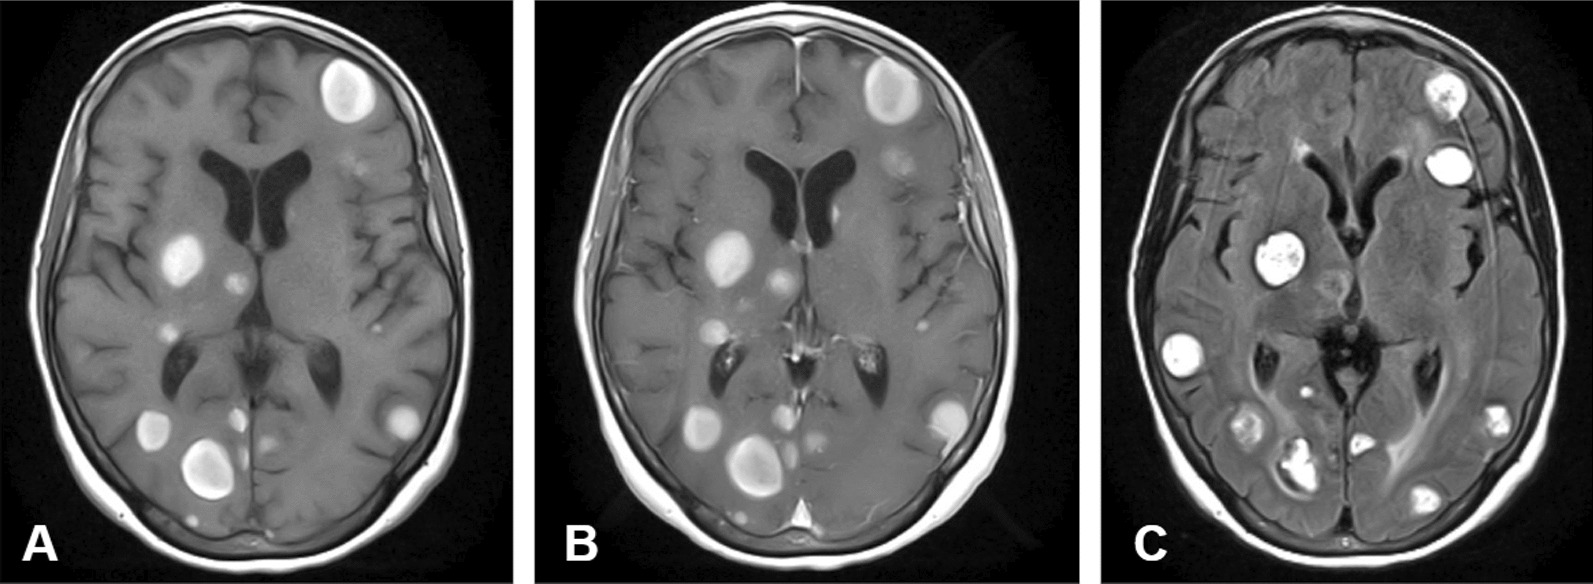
Magnetic resonance image brain axial slices at the level of the hypothalamus demonstrating innumerable round, bilateral lesions consistent with florid metastatic spread. A T1-weighted without contrast. B T1-weighted with contrast. C T2-fluid attenuated inversion recovery imaging demonstrating vasogenic edema of occipital lesions
